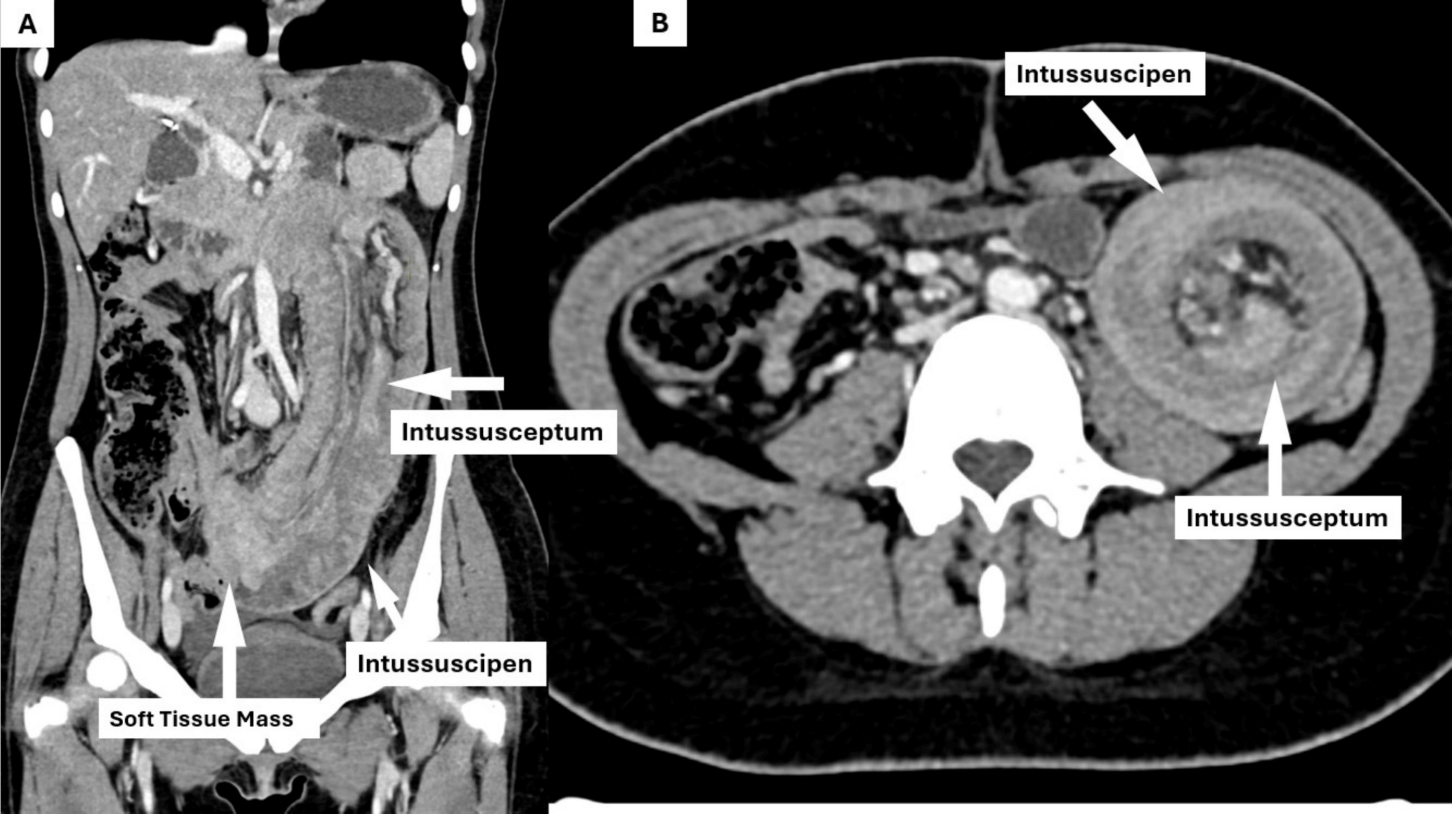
CT scout films showing the intussusceptum, intussuscipen and the soft tissue mass (leading point) (A) Coronal CT image demonstrating a long segment of small bowel intussusception. The intussuscipiens and intussusceptum are labeled, along with a soft tissue mass acting as the lead point. (B) Axial CT image demonstrating the characteristic “target sign” of intussusception, with the intussuscipiens forming the outer bowel wall and the intussusceptum visible centrally.
